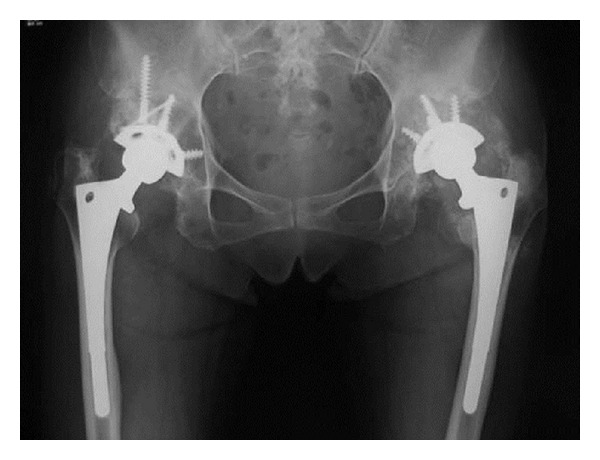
X-ray findings at the initial diagnosis.
